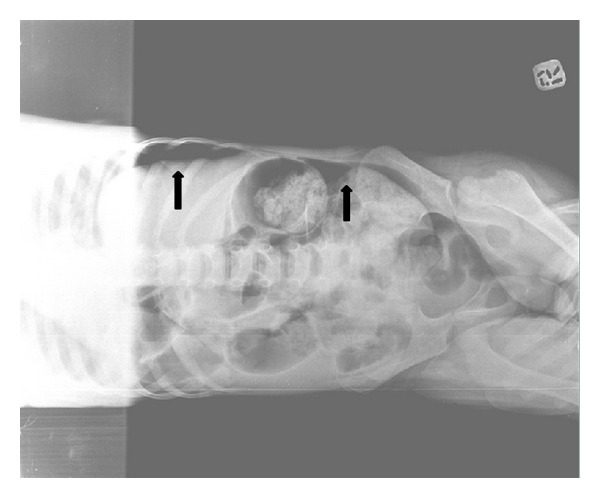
Lateral decubitus radiograph shows air movement within abdominal cavity.
